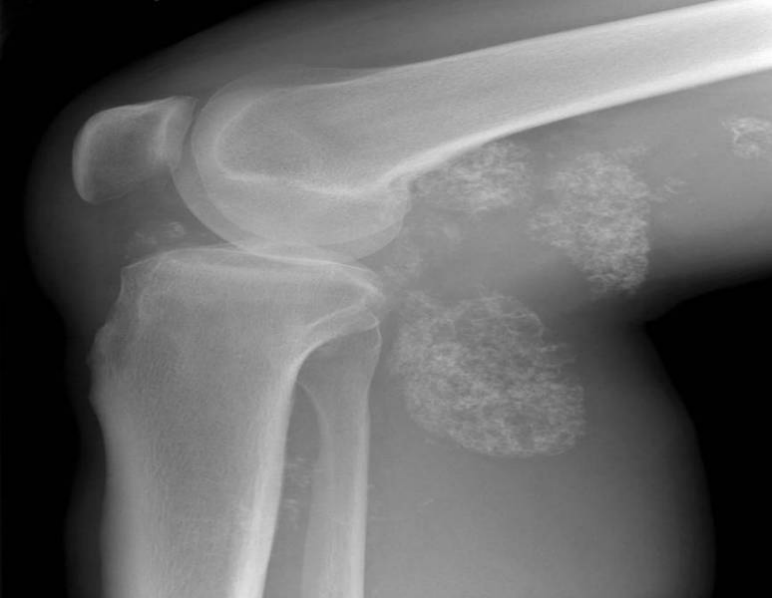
Plain radiograph and magnetic resonance imaging scans showing multiple soft tissue calcifications within and outside the joint capsule of the right knee.
